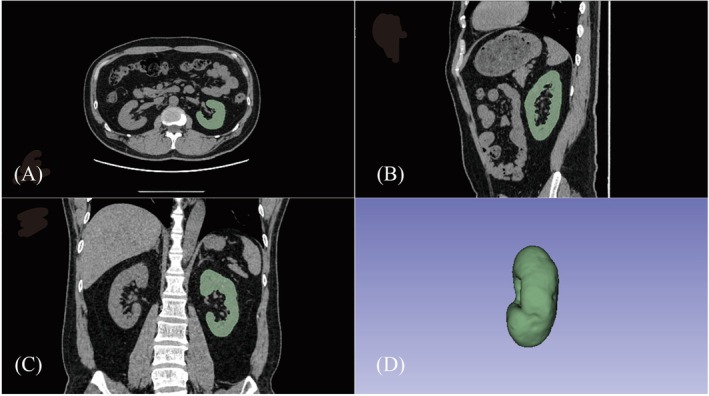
Using 3D Slicer for automatic kidney segmentation. (A)–(C) represent the transverse, sagittal and coronal planes of the CT scan, respectively; (D) is the image after three‐dimensional reconstruction.
